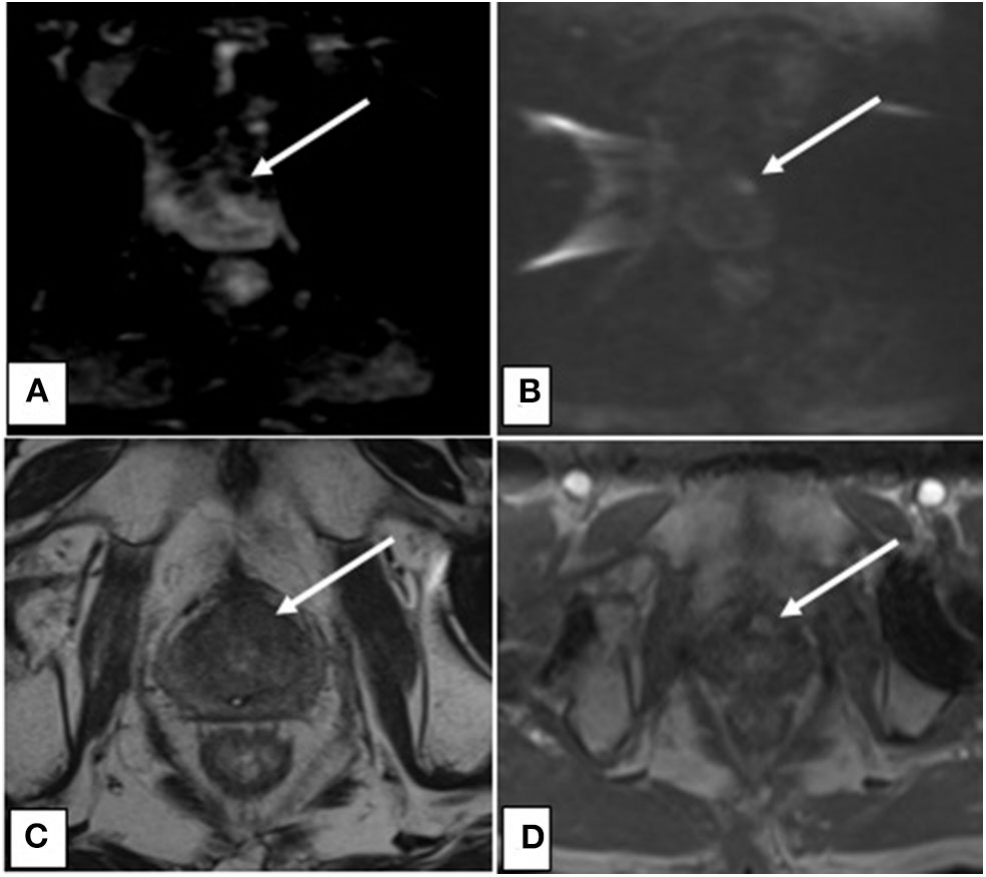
(A) Axial ADC map showing decreased signal intensity associated with lesion indicative of restricted diffusion. (B) Axial DWI showing increased signal intensity associated with lesion indicative of restricted diffusion. (C) Axial T2 showing decreased signal intensity associated with the lesion. (D) Axial DCE with contrast showing increased perfusion to the lesion.
